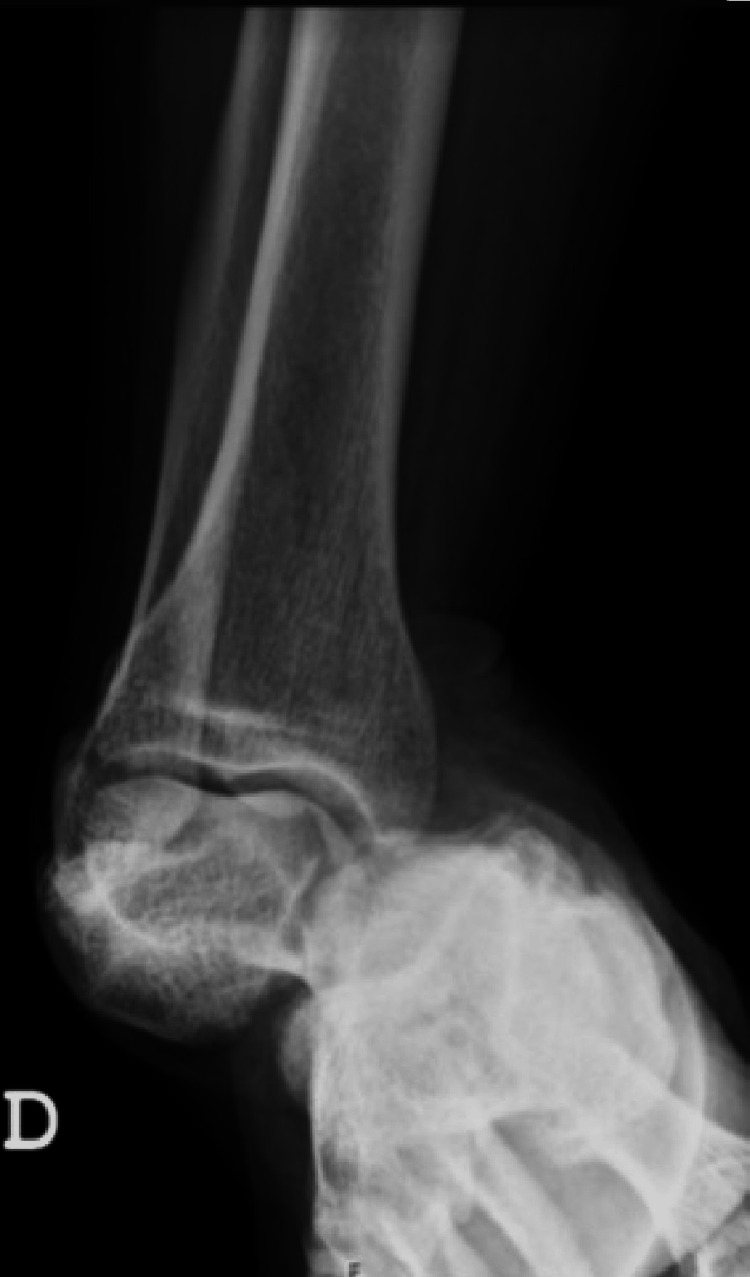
Anteroposterior radiographic view showing dislocation of the talonavicular and talocalcaneal joints without fracture.
